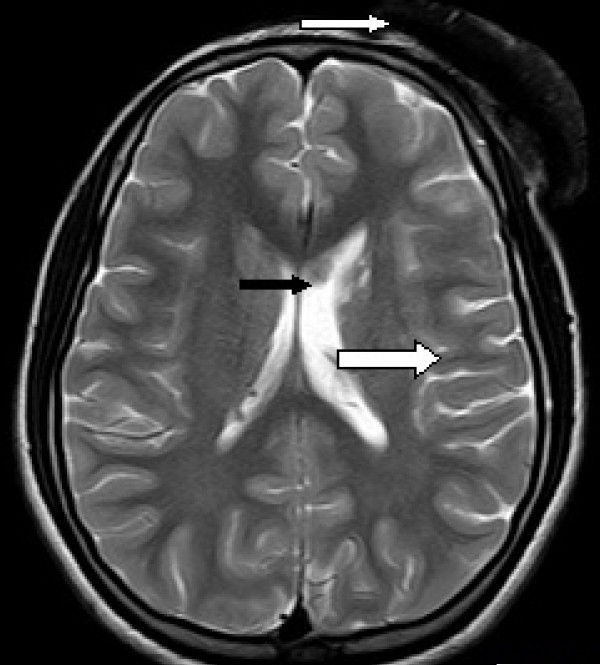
Magnetic resonance imaging findings. These MRI scans show a single 8.99 cm × 2.26 cm abnormal signal intensity involving the scalp in the left frontoparietal region appearing isointense to hyperintense on T1-weighted images (thin white arrow) with few calcified subependymal nodules within the body of the lateral ventricles on the right side (black arrow), a few small tubers with cortical dysplasia (thick white arrow) in the left frontoparietal region, and left maxillary sinusitis.
